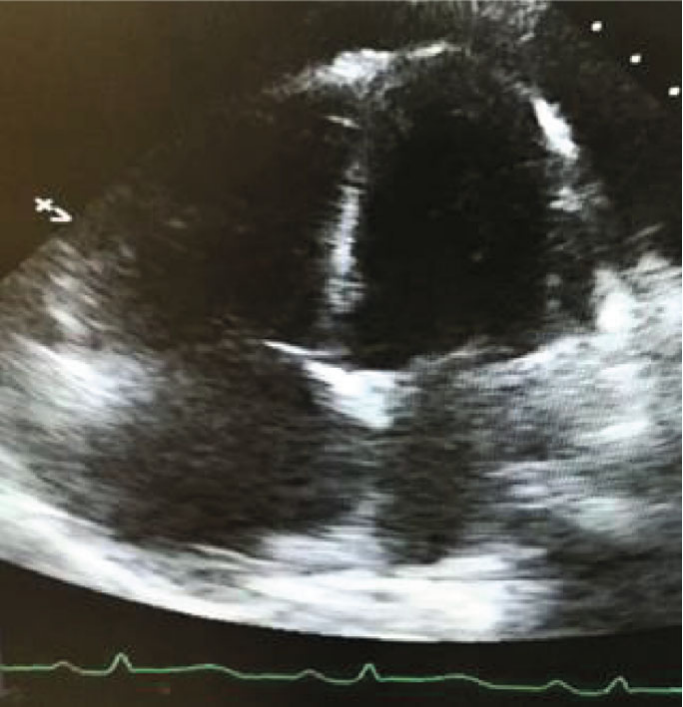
Transthoracic echocardiogram showing marked dilatation of right ventricle with globally reduced right ventricular systolic function.
